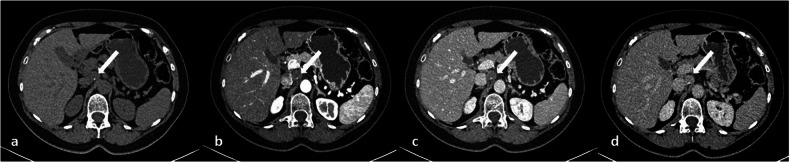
a–d CT images in a 64-year-old woman with a history of breast cancer incidentally reveal a round, paracaval solitary mass (white arrows); in the non-contrast-enhanced image (a) the mass appears solid and isodense with small, focal, inner calcification; after contrast medium administration, progressive enhancement is visible (b–d)
